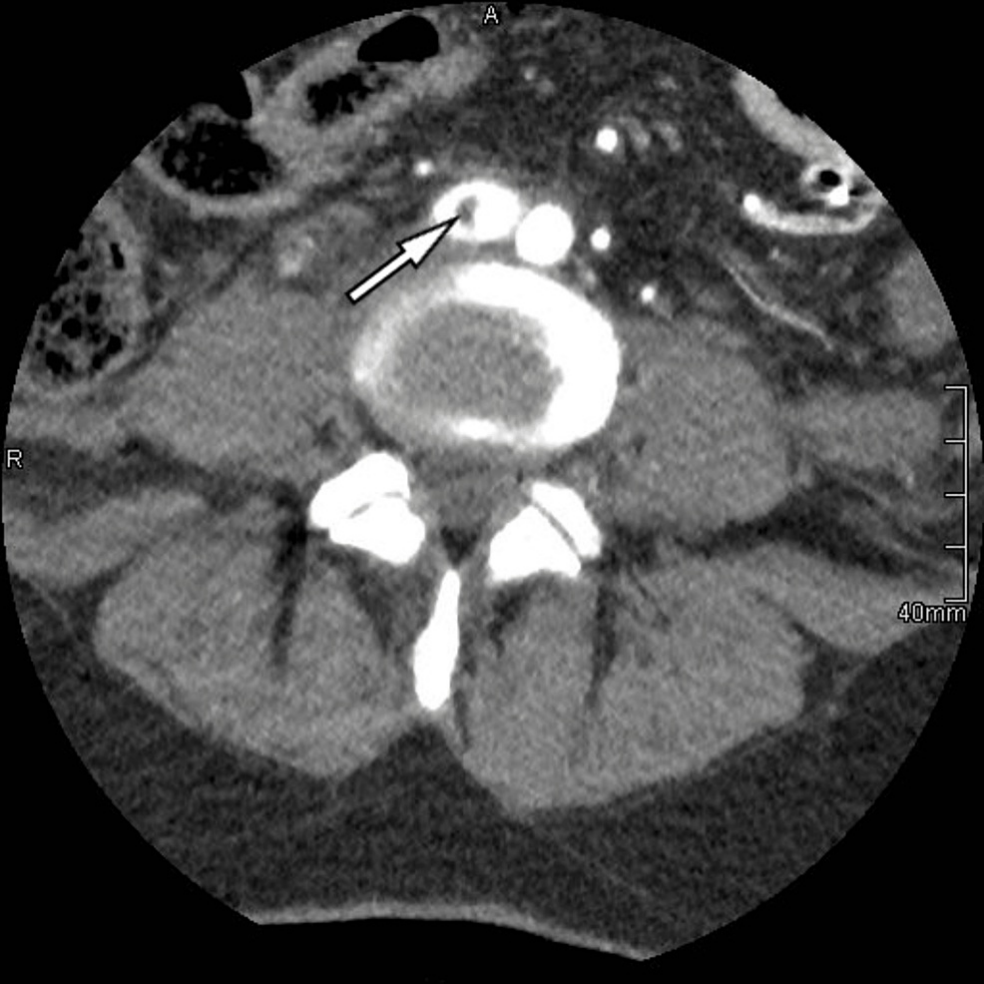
Computed tomography angiography after surgery, axial image, partial thrombosis of the right iliac artery (arrow)
